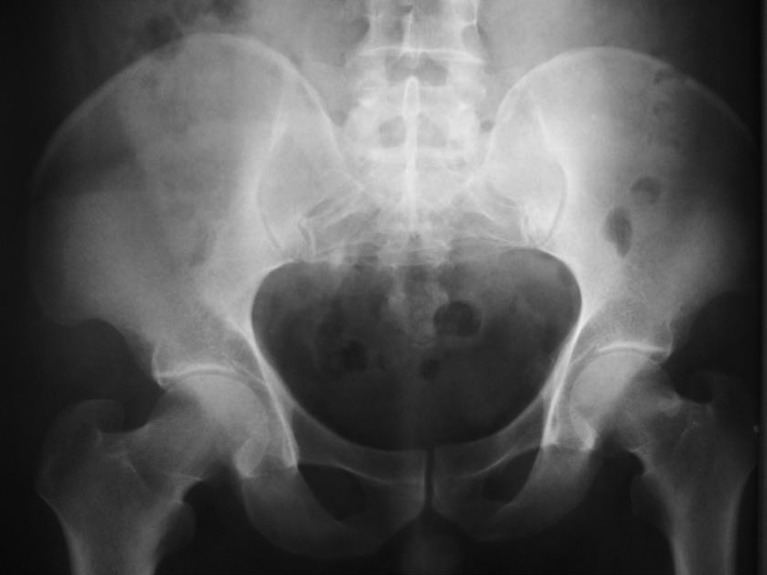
Postoperative pelvic X-ray results of a patient (case 1) with bladder diverticulum accompanied by bladder calculus.
